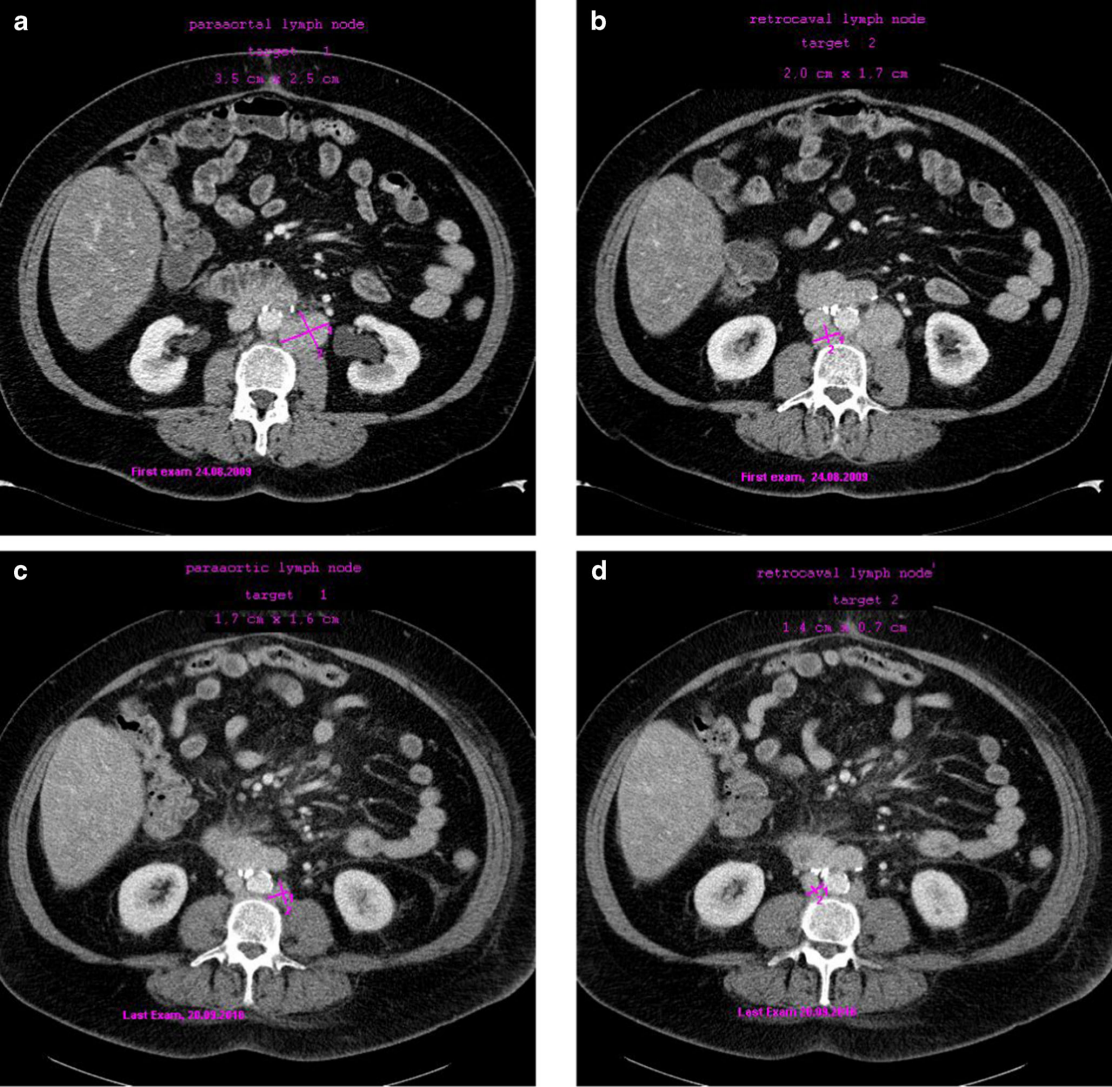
CT scans of a patient with cervical cancer who experienced a confirmed PR. a and b: baseline scans; c and d: following 14 cycles of treatment with BI 831266. PR was first documented after cycle 10. *PR* partial response
